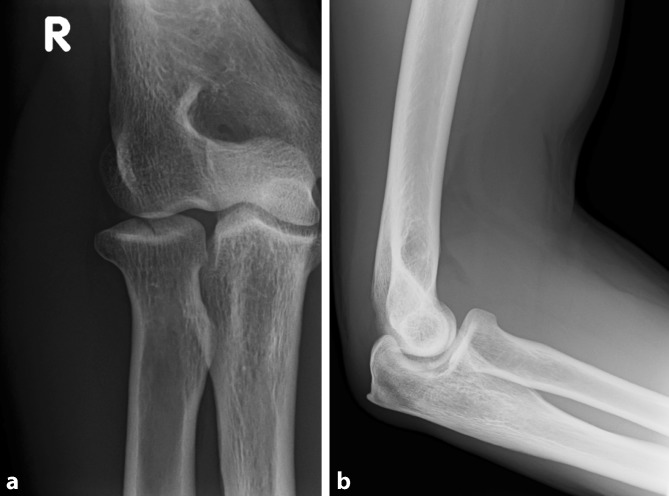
Radiographs (two planes) of a radial head fracture (Mason I)
